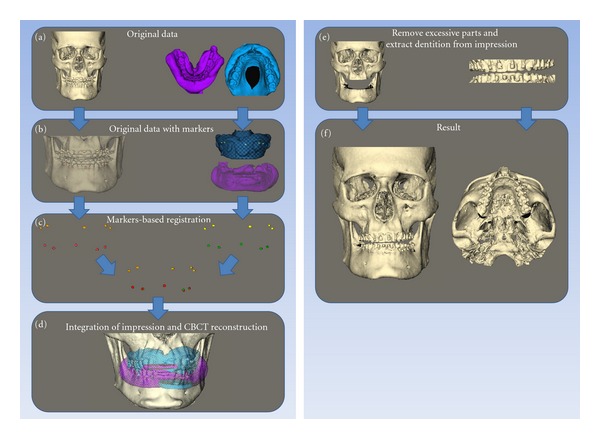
Matching procedure of the digital dental casts in the CBCT: (a) original datasets with 3D reconstruction patient scan and impression scan; (b) original datasets with markers extracted; (c) marker based registration; (d) integration of the reconstruction of the impression scans and CBCT reconstruction of the patients scan; (e) removal of excessive parts from the CBCT and extraction of dentition from the impressions; (f) final result.
